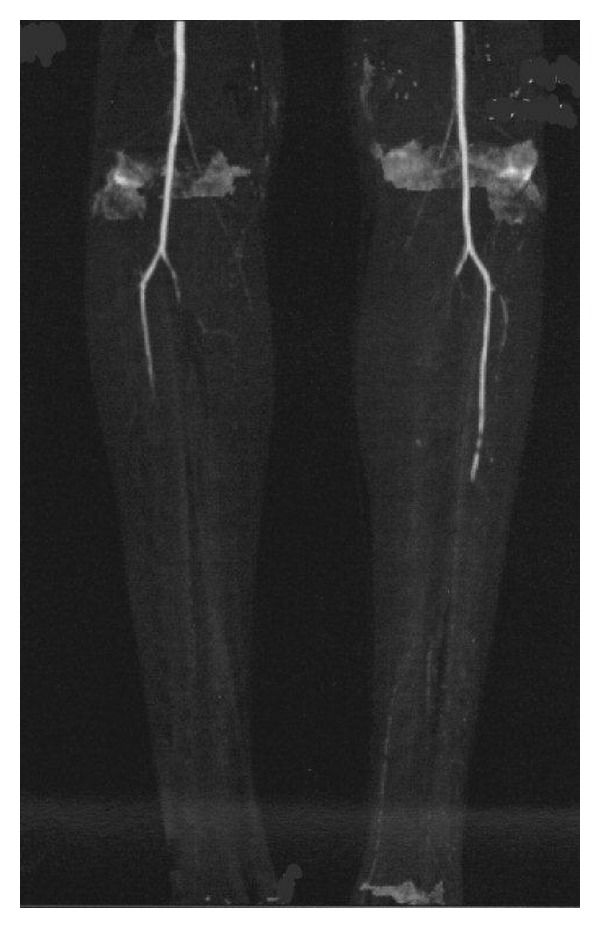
CT angiography of the lower limbs showing distal posterior and anterior tibial artery occlusion of the left side and three-vessel occlusion of the right side.
